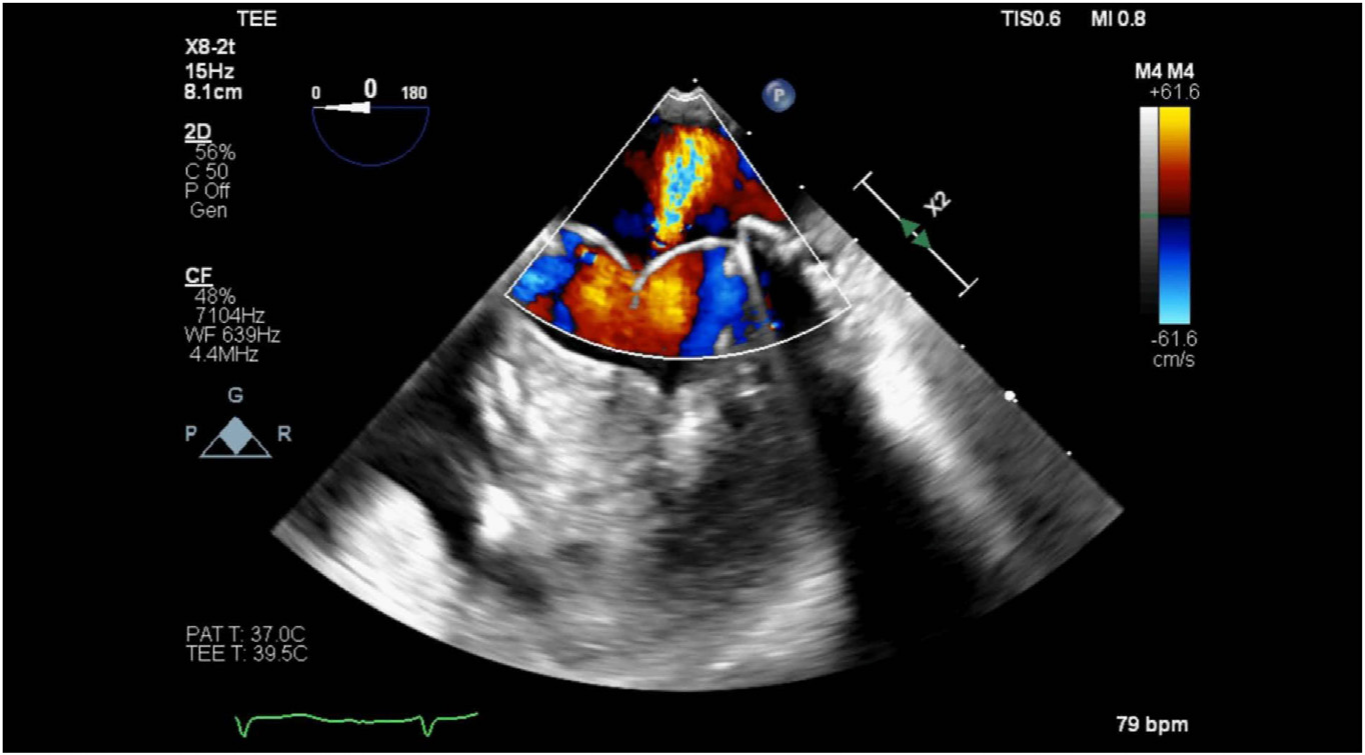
Two-dimensional TEE, midesophageal 4-chamber view (foreshortened) with color-flow Doppler, systolic phase, demonstrates MR.
